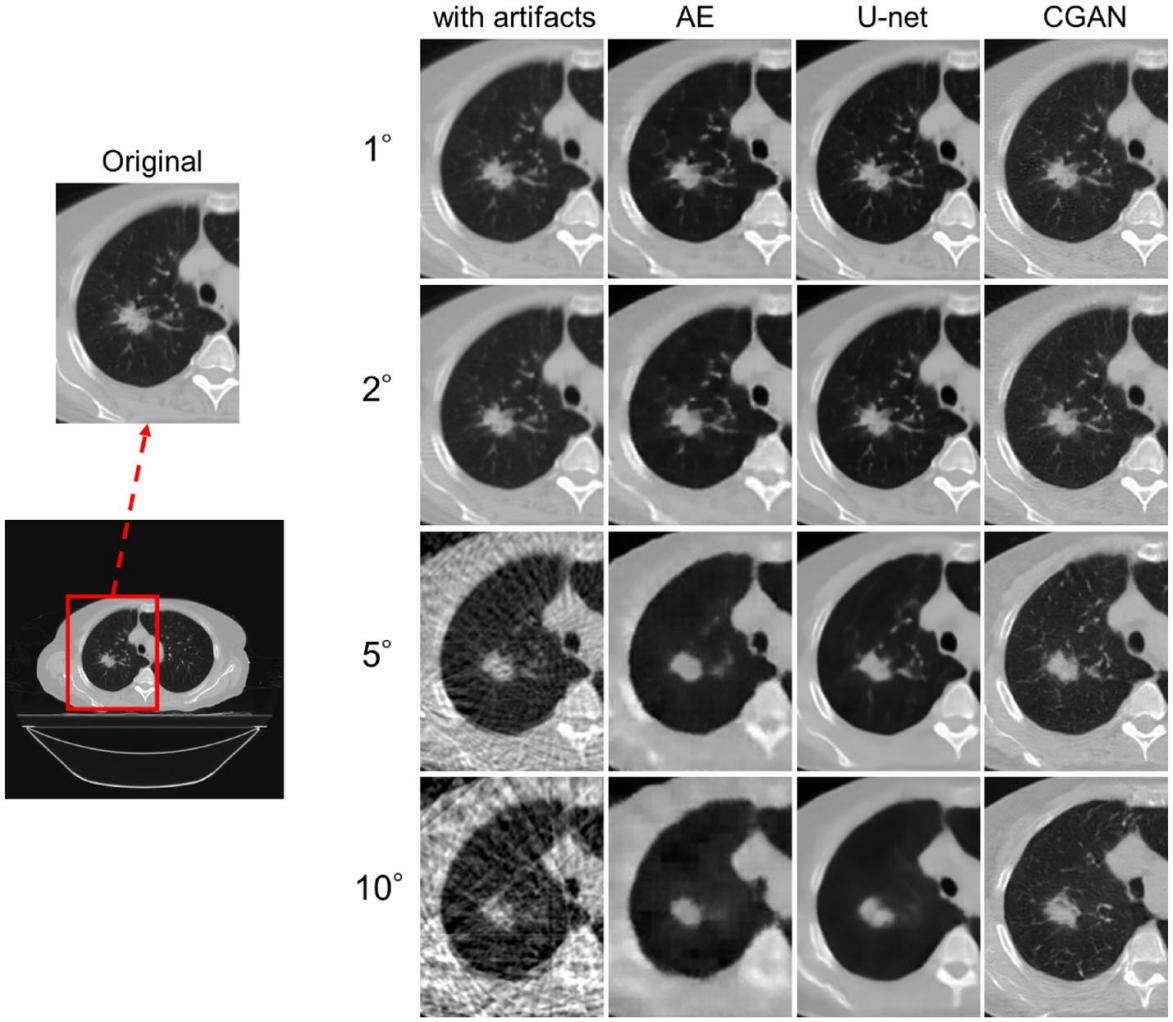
Enlarged images of the region indicated by the red region of interest with each result of sparse-view CT. All images are shown with the same window width and levels.
